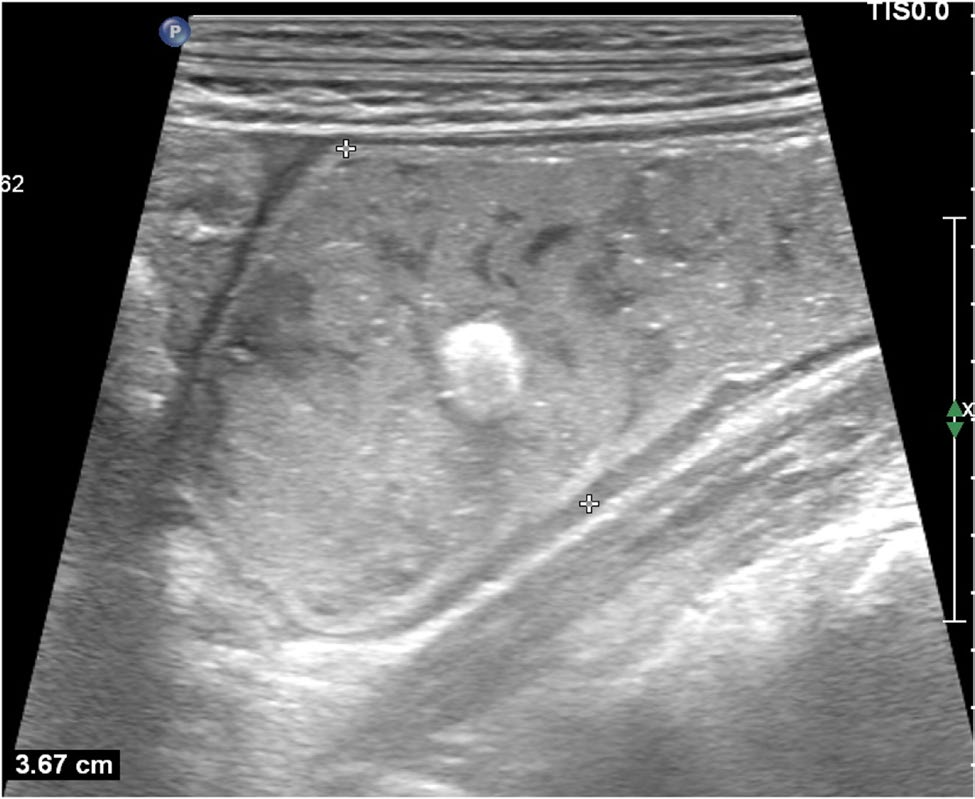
High-frequency ultrasound shows the dilated small intestine with an inner diameter of 3.7 cm
